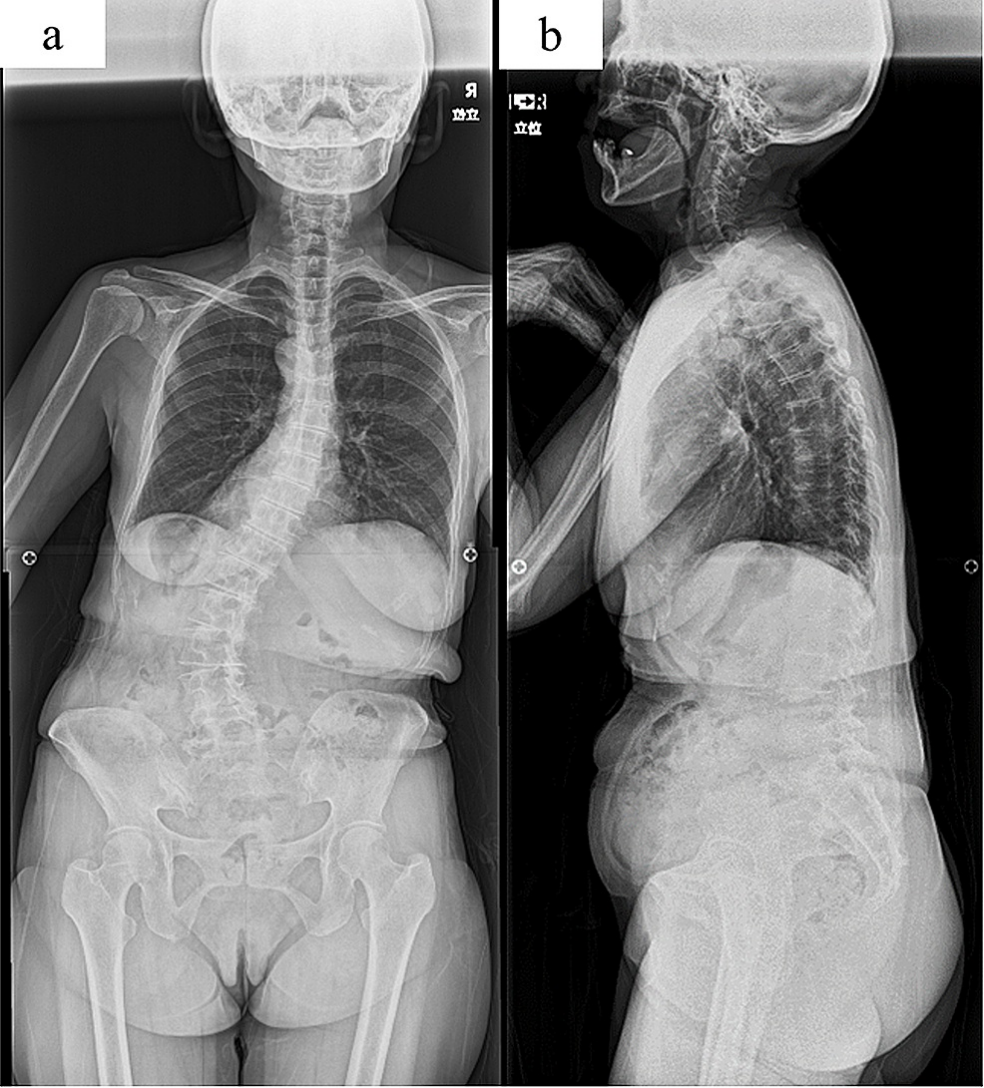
Preoperative images Preoperative posterolateral (a) and lateral (b) standing radiographs showing degenerative lumbar scoliosis and kyphotic deformities with poor global alignment.
